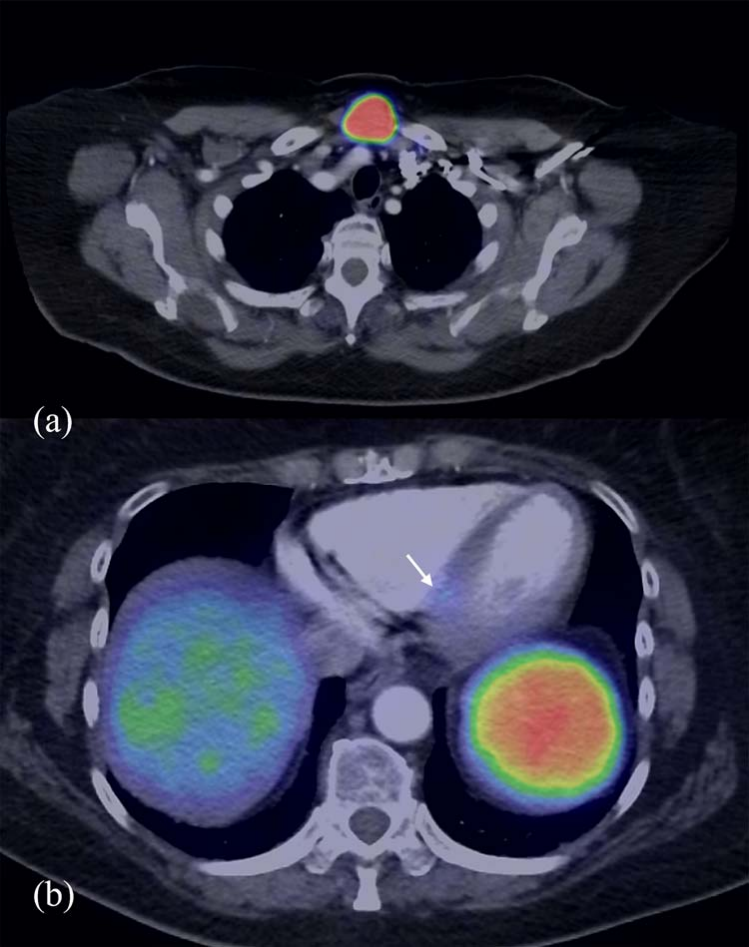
DOTA PET/CT demonstrating avidity in the midline neck mass (a) and the left ventricular septum inferiorly (b).
